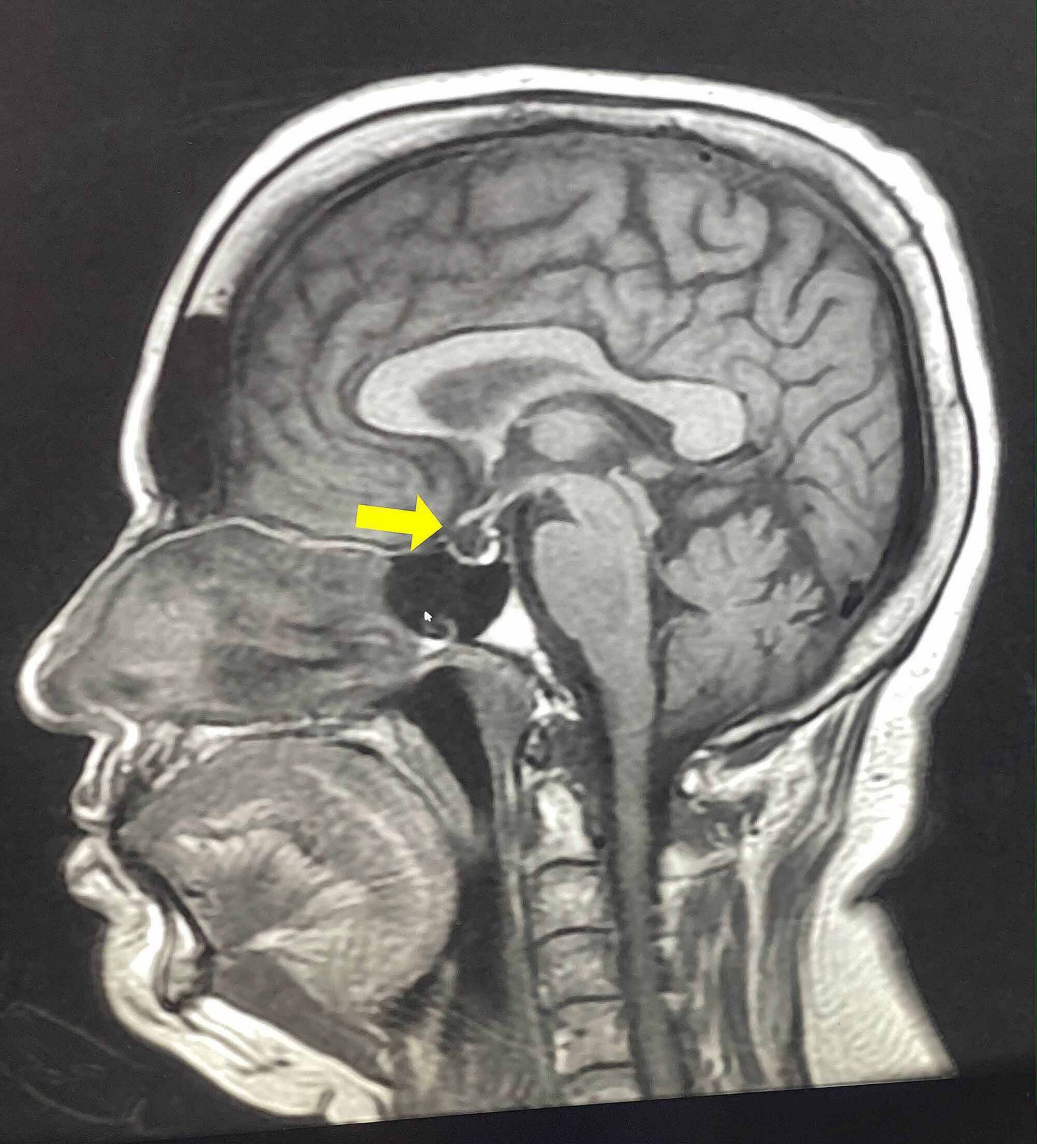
Sagittal MRI view of the hypothalamopituitary showing partially empty sella (yellow arrow)
